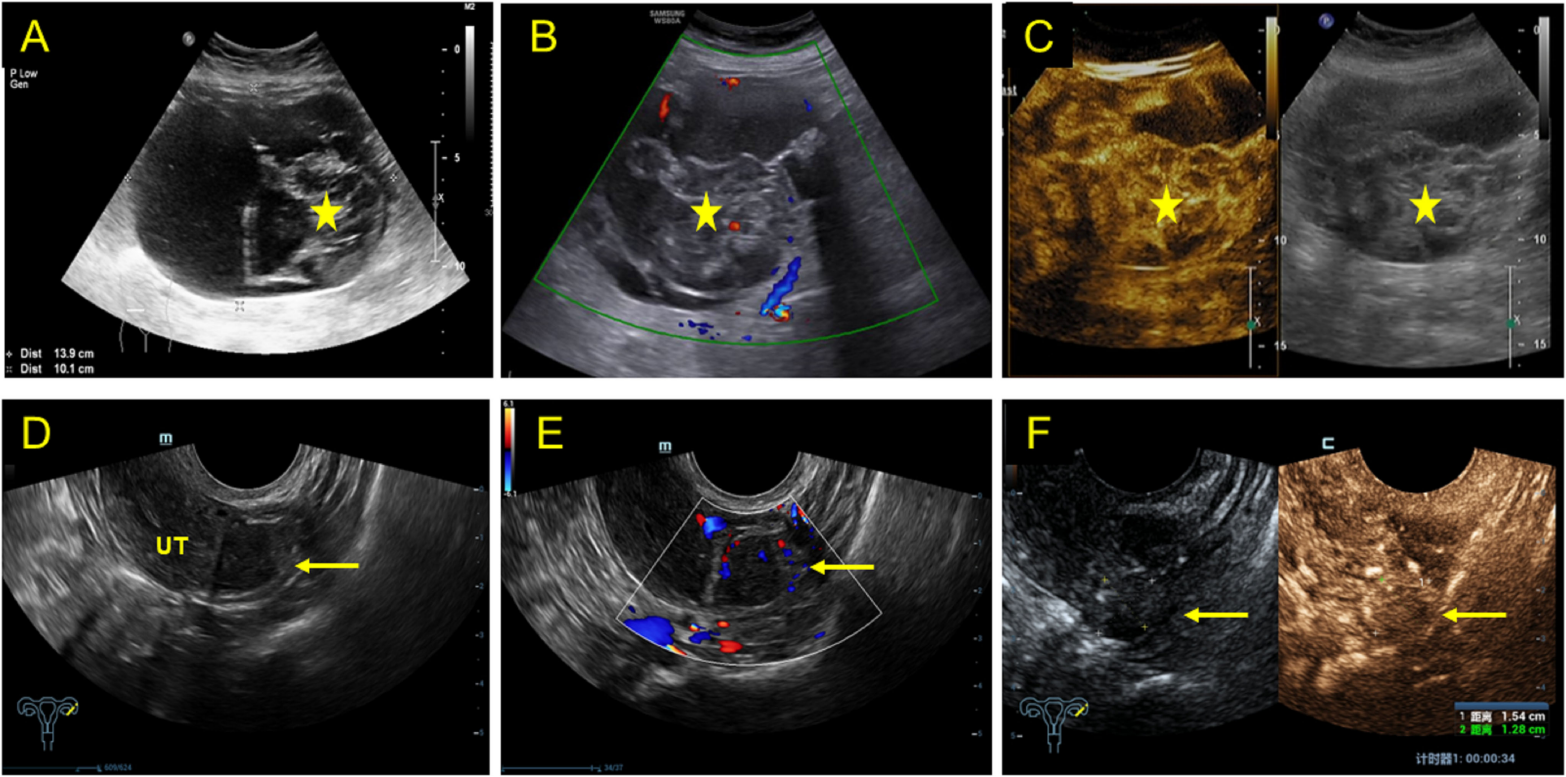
2D, CDFI, and CEUS characteristics in a mixed cystic and solid lesion (upper row) and a solid lesion (lower row). (A) 2D transabdominal ultrasound showing a mixed cystic and solid (asterisk) lesion in the right adnexal area with a diameter of 13.9 × 10.1 cm. (B) Rich blood flow was detected in the protrusions and solid part. (C) Transverse abdominal contrast-enhanced ultrasound showing the lesion significantly enhanced at 15 s after contrast agent injection and then slowly washed out. (D) Transverse transvaginal sonography showing a solid lesion (arrow) in the left ovary with a diameter of 1.70 × 1.34 cm. (E) Color Doppler image showing rich blood flow at the inner side of the solid lesion. (F) Transverse transvaginal contrast-enhanced ultrasound demonstrating that the solid lesion of the left adnexal area began showing equal enhancement at 24 s after contrast agent injection and peaked at 42 s.
